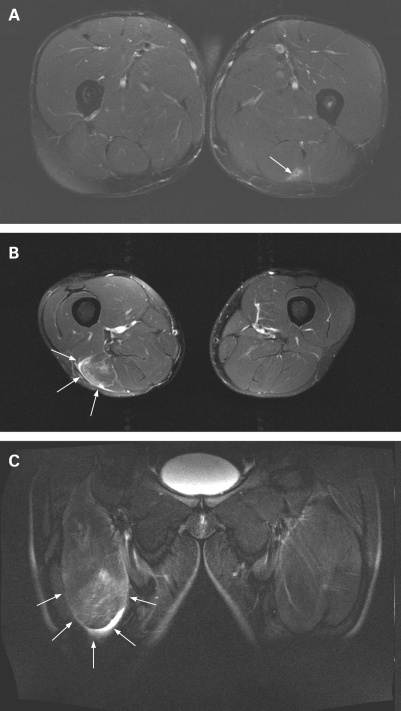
Magnetic resonance images of different grades of muscle lesion: (A) grade I lesion; (B) grade II lesion; (C) grade III lesion.
